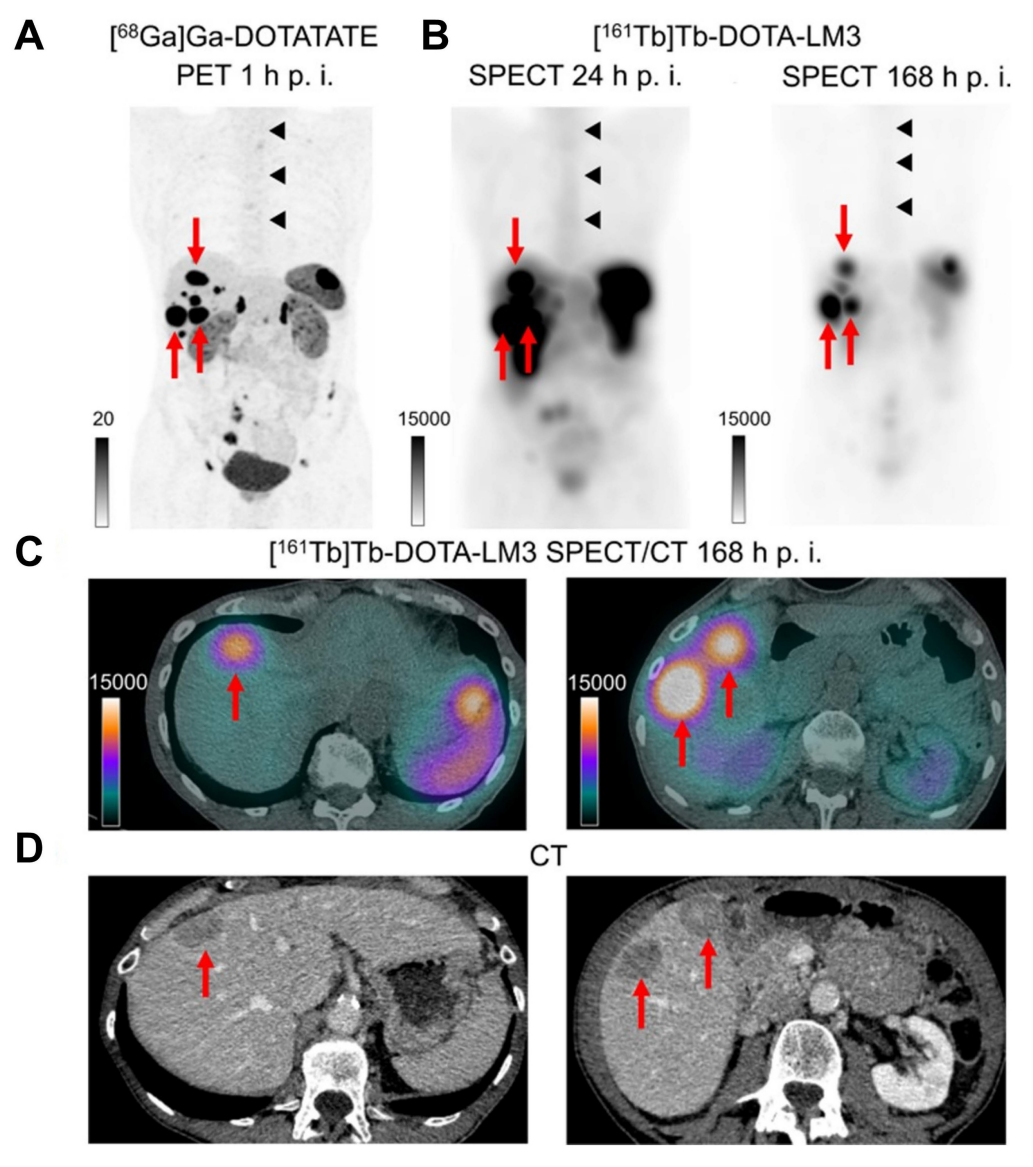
First-in-human administration of [^161^Tb]Tb-labeled SSTR-2 antagonist ([^161^Tb]Tb-DOTA-LM3) in a patient with a metastatic NET of the ileum. (A) Representative PET imaging after 1 h post-administration of [^68^Ga]Ga-DOTATATE. (B) Representative SPECT imaging after 24 and 168 h post-administration of [^161^Tb]Tb-DOTA-LM3. (C) Transverse SPECT image 168 h post-injection of [^161^Tb]Tb-DOTA-LM3 and correlated CT image (D). The accumulated tumor area signal was indicated by the *red arrow*. Copyrights© 2024 Springer Nature.
